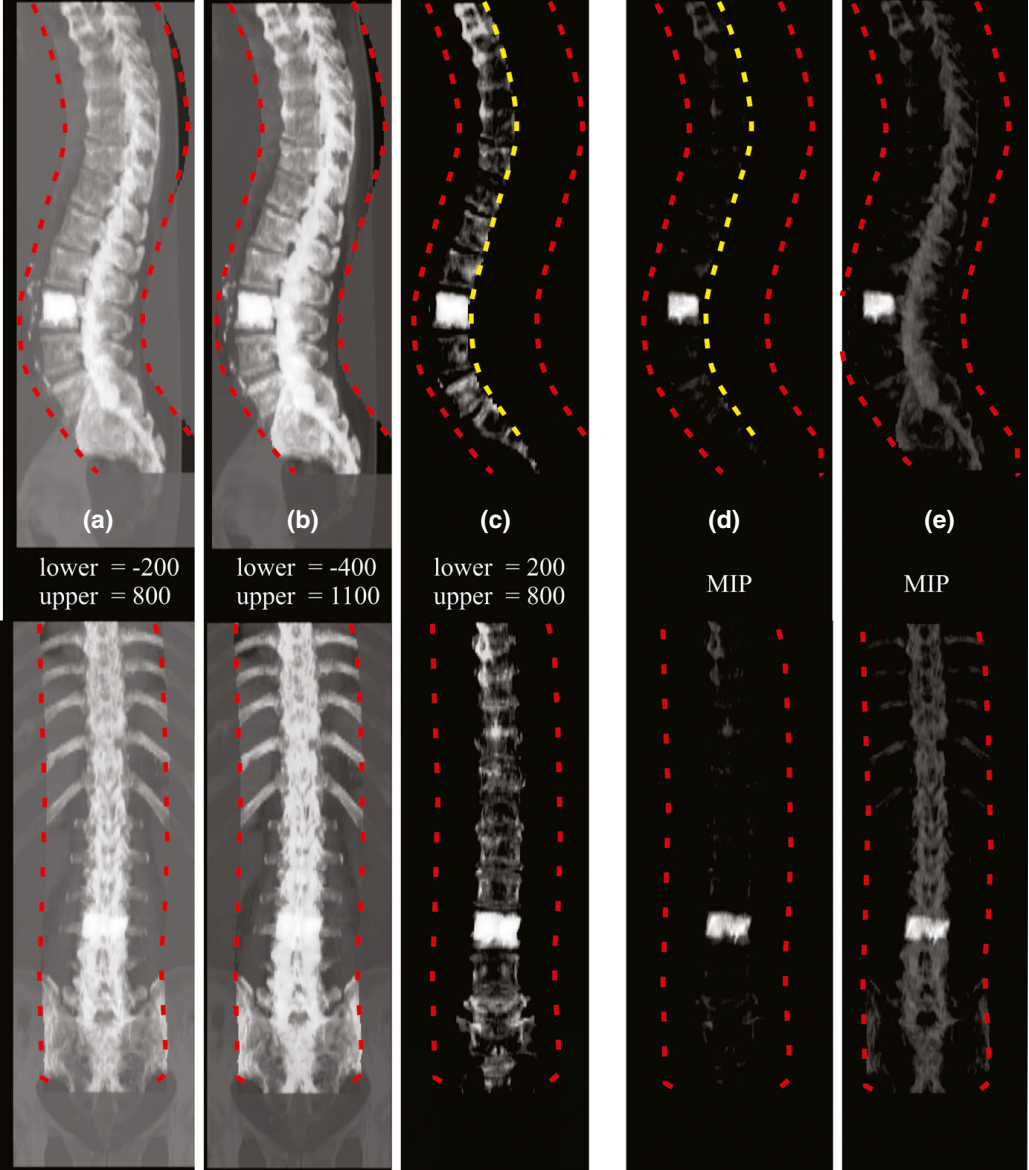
CIP and MIP image pairs. Custom (a–c) and maximum (d–e) intensity projection image pairs depicting sagittal (top row) and coronal (bottom row) views. “Lower” and “upper” indicate bounds for CT number normalization before application of CIP formation formula in 2.C.1. Red dashed lines indicate VOI boundaries formed from the spinal canal. Yellow dashed lines indicate the boundary further constraining the VOI at the midpoint of each axial slice from the spinal canal (thus excluding posterior processes from the VOI). In this patient, from the cross‐validation dataset, a lumbar vertebroplasty is present and creates a high‐intensity region in the IP images. [Color figure can be viewed at wileyonlinelibrary.com]
